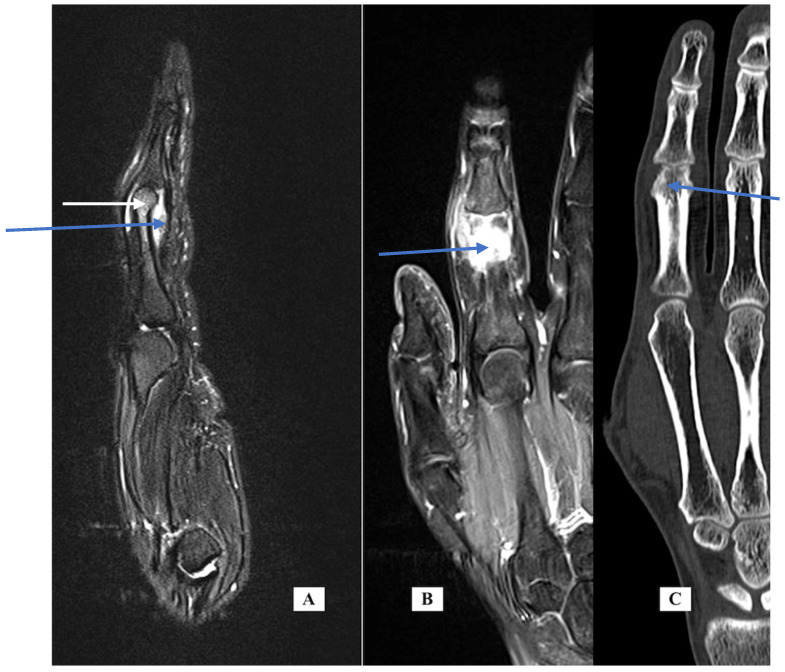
Case 2—MRI of the finger, sagittal view (A) demonstrating synovitis (blue arrow) and bone marrow edema of distal P1 (white arrow); coronal view (B); CT scan of the finger, coronal view (C) demonstrating marginal erosion at the radial collateral ligament origin.
